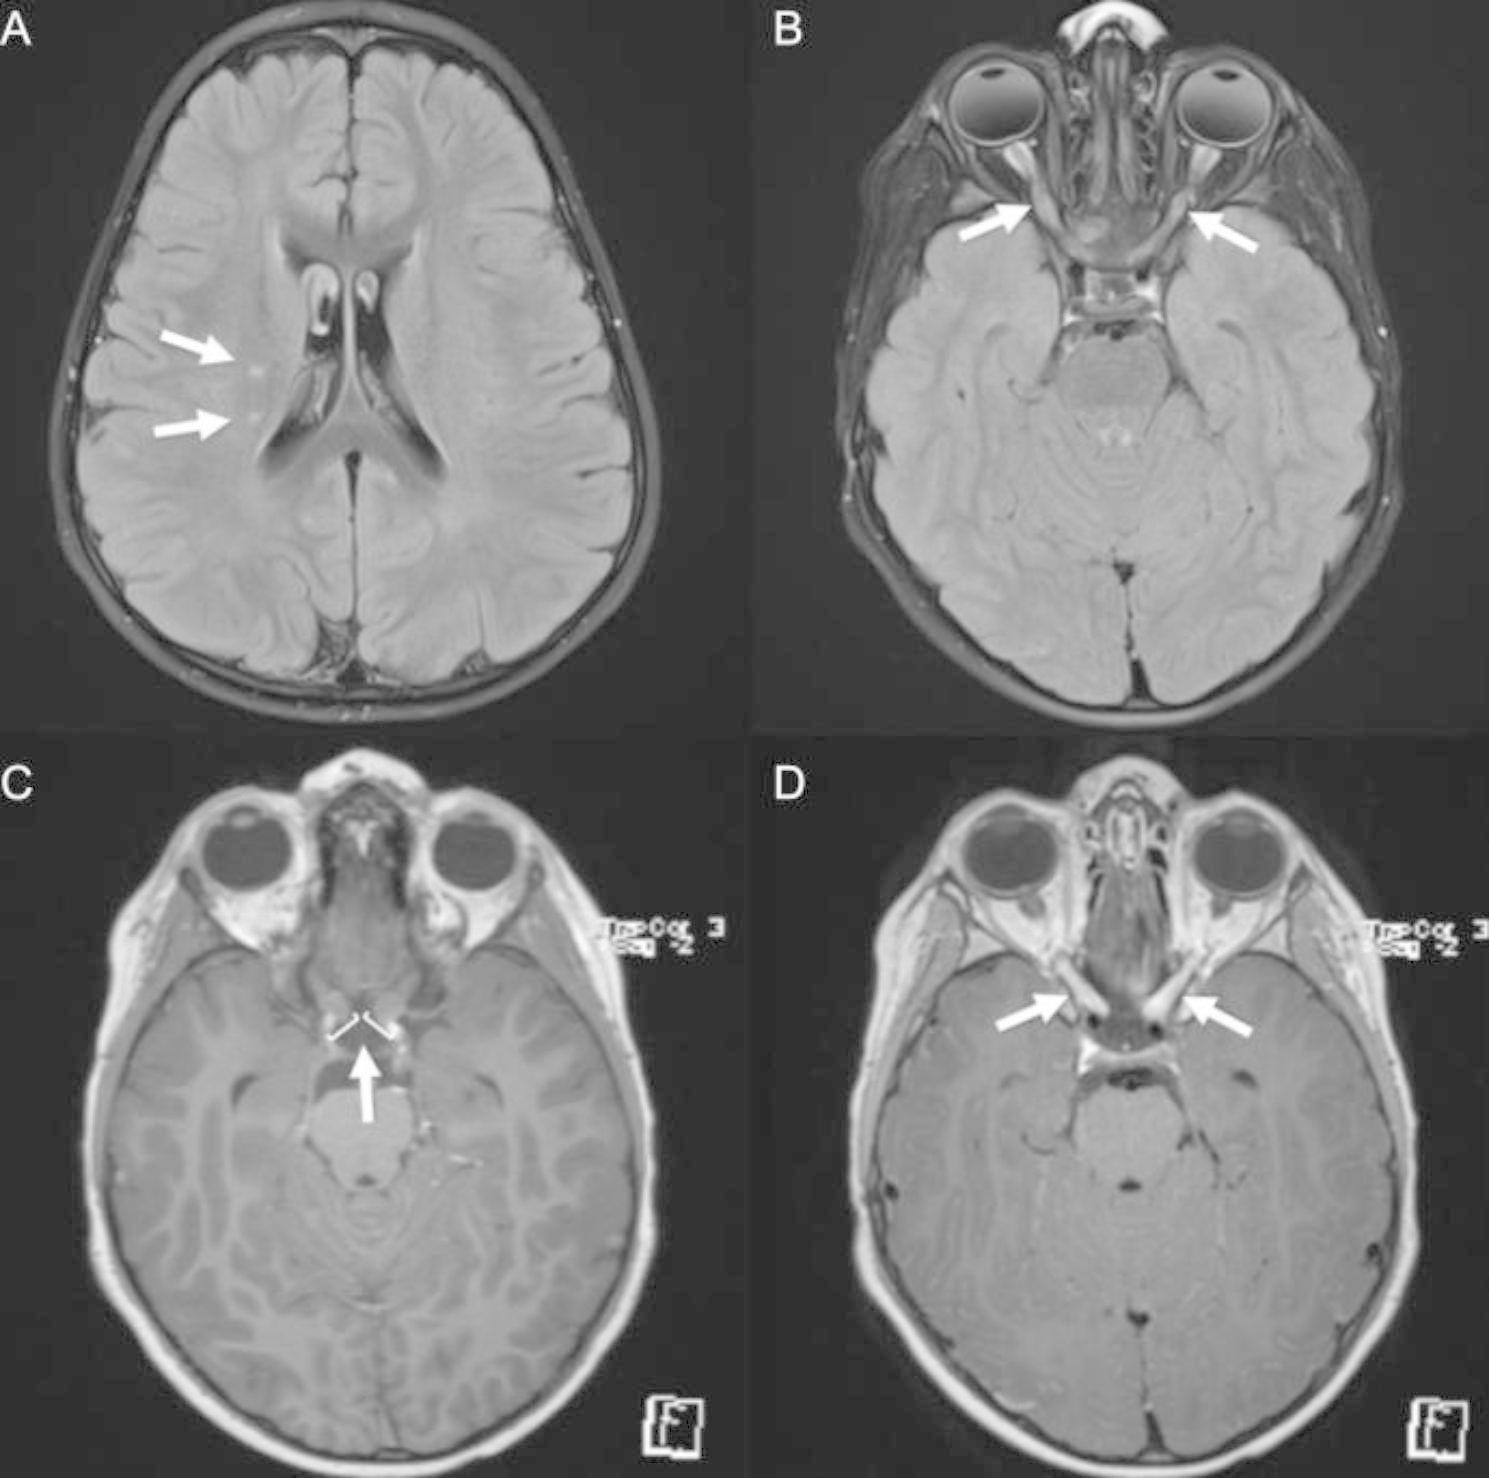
MRI brain images from initial hospitalization. Axial fluid-attenuated inversion recovery (FLAIR) sequences demonstrated (A) two small T2/FLAIR hyperintense 3-4 mm foci in the right frontal white matter and (B) T2/FLAIR hyperintense bilateral optic nerves. Axial T1 post-gadolinium images demonstrate (C) thickening of the optic nerves to 5–7 mm with (D) marked enhancement of the nerve sheaths consistent with bilateral optic neuritis
